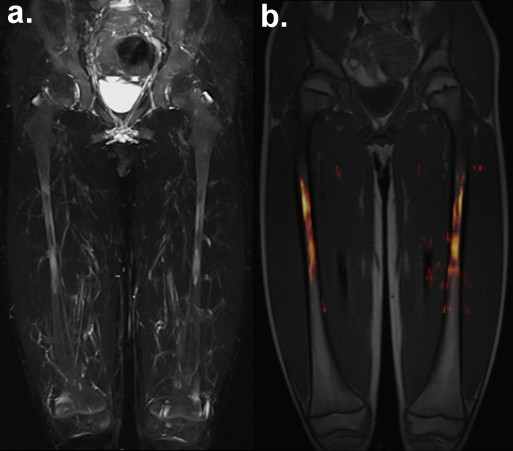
Visual presentation of femoral bone marrow oedema with T2W TIRM and DWI. Symmetrical bone marrow oedema of the femoral diaphysis in an 8-year-old boy with CNO. Visualisation with maximum intensity projection (MIP) of coronal T2W TIRM (a) and 3D image fusion of colourised transversal DWI b = 800 overlay on coronal pre-contrast T1W TSE (b).
